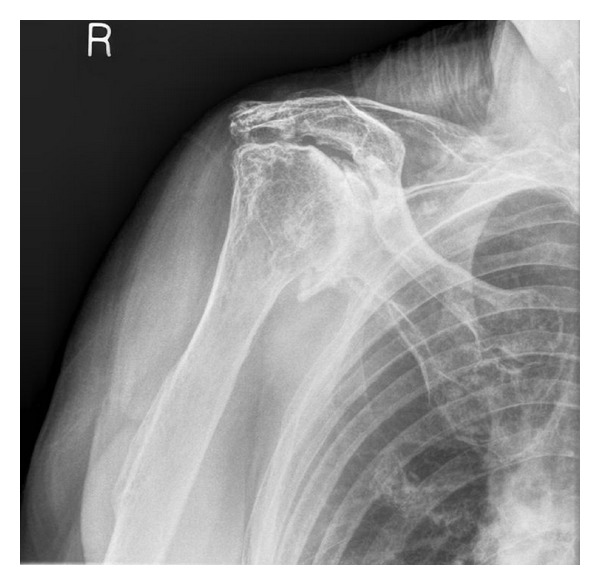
AP radiograph of the right shoulder taken preoperatively.
